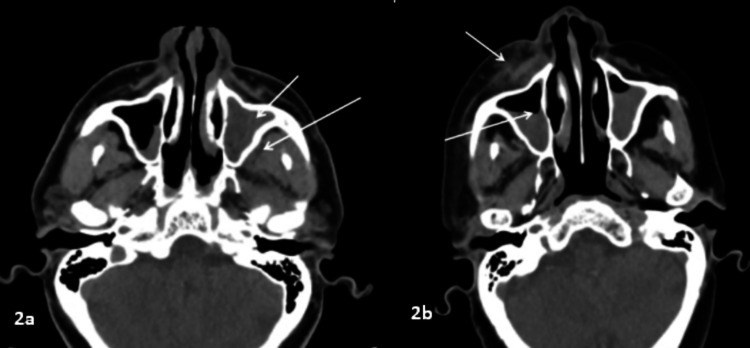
Periantral involvement in different patients 2a: Axial CT scan of a 52-year-old patient with mucormycosis showing complete sinus opacification of the left maxillary sinus (short arrow) with retroantral fat stranding (long arrow). 2b: Axial CT scan of a 57-year-old patient with mucormycosis showing air-fluid level in the right maxillary sinus (long arrow) with preantral fat standing (short arrow). Both images show periantral involvement with an intact bony wall, suggestive of the perineural spread of the infection. CT: computed tomography
